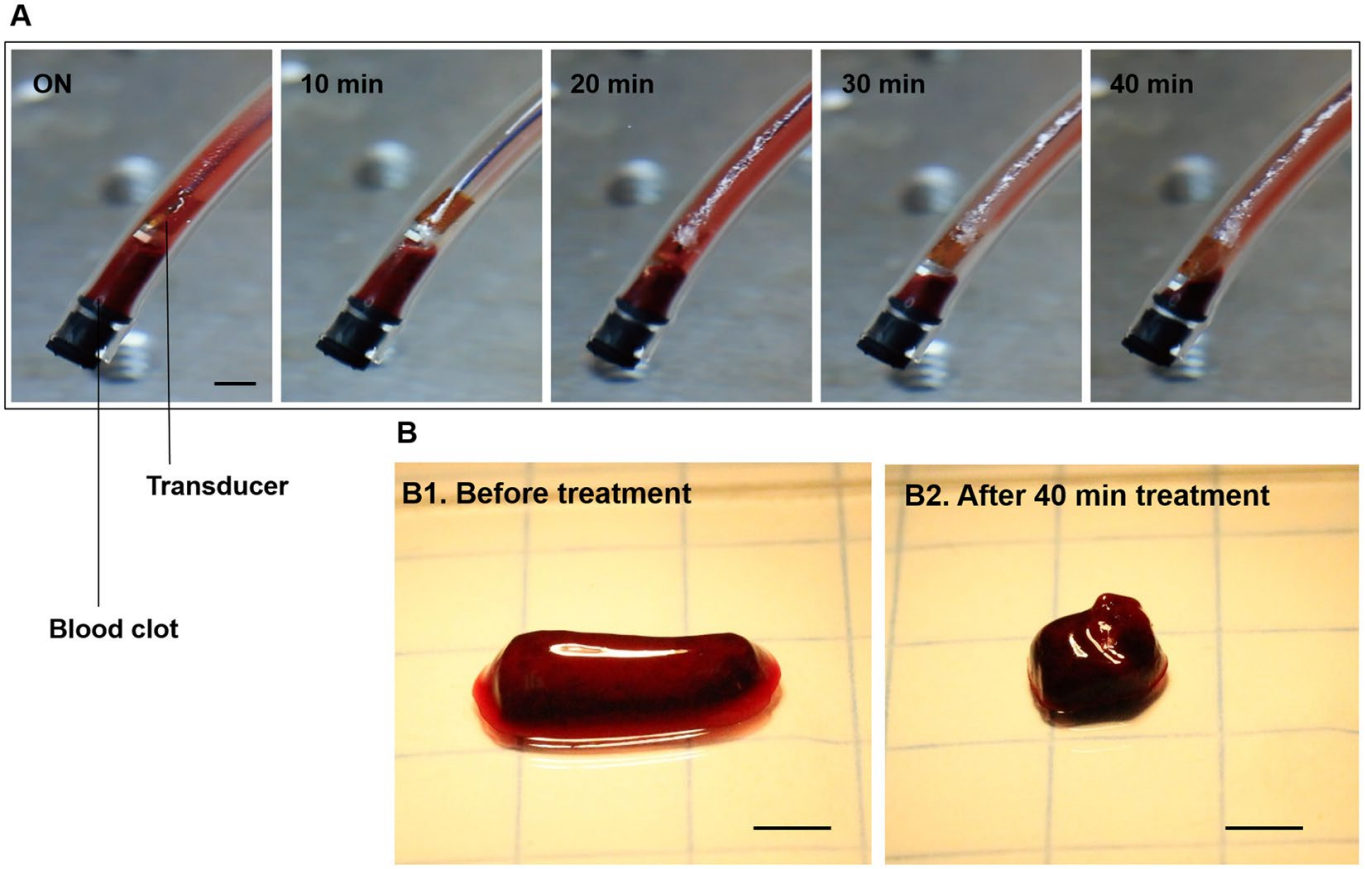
In vitro* clot lysis by microbubble-mediated intravascular ultrasound. (A) Captured images during 40 min treatment by using the planar-aperture prototype (input conditions of 620 kHz, 80 V_pp_, 10% duty cycle with 100 μl/min injection of 50^8^ bubbles/ml). A smaller (120 mg) clot was used for monitoring the volume reduction (scale bar = 3 mm). Due to the high bubble concentration (50^8^ bubbles/ml) we observed that the undestroyed bubbles were attached to the transducer and the inner surface of the tygon tube. (B) A 120 mg clot was reduced to 50 mg after the 40 min treatment (scale bar = 3 mm).
